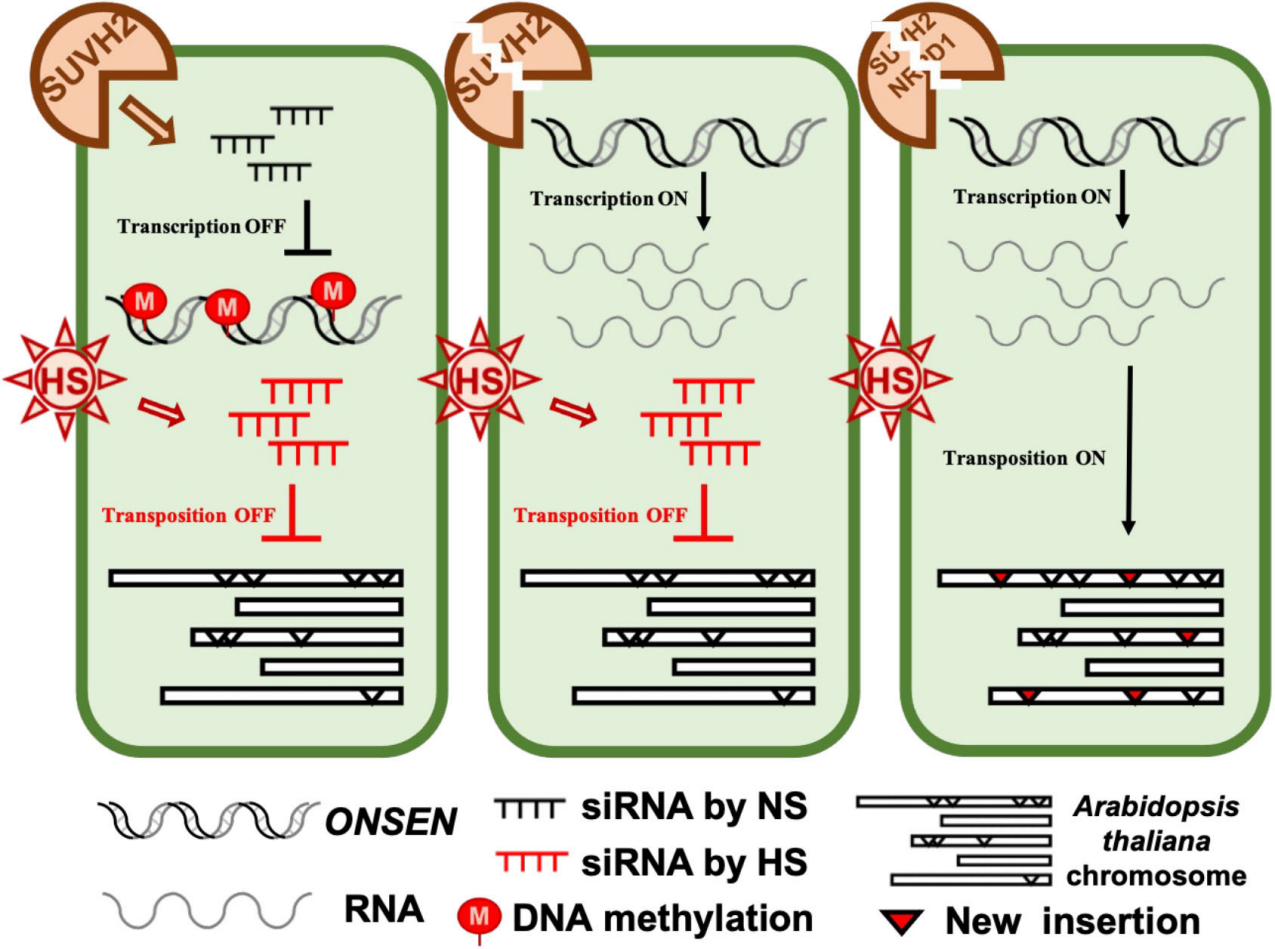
The model of SUVH2 involves *ONSEN* regulation. In the wild-type, intact RdDM can produce siRNAs to suppress *ONSEN* transcription. In the *suvh2* mutant, the RdDM pathway is disrupted, releasing *ONSEN* transcription by heat stress. Under heat stress, plants bypass the SUVH2-dependent pathway to produce new siRNAs, and these siRNAs produced by heat stress prevent *ONSEN* from transposing into other sites on the chromosome. In the *suvh2/nrpd1* double mutant, siRNA could not be synthesized under heat stress conditions releasing of transpositional silencing of *ONSEN*.
